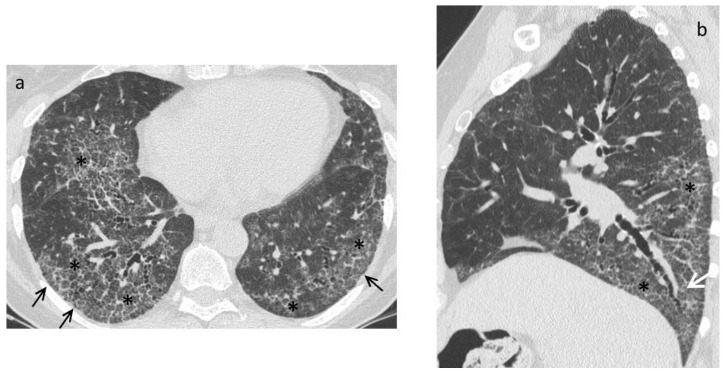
Late stage. High resolution CT images in axial (a) and sagittal (b) plane show a fibrotic nonspecific interstitial pneumonia (NSIP) pattern characterized by the presence of bilateral extensive ground glass opacities (*) with a peripheral and symmetric distribution with signs of fibrosis due to the presence of reticulations (black arrows) and traction bronchiectasis (white arrows).
